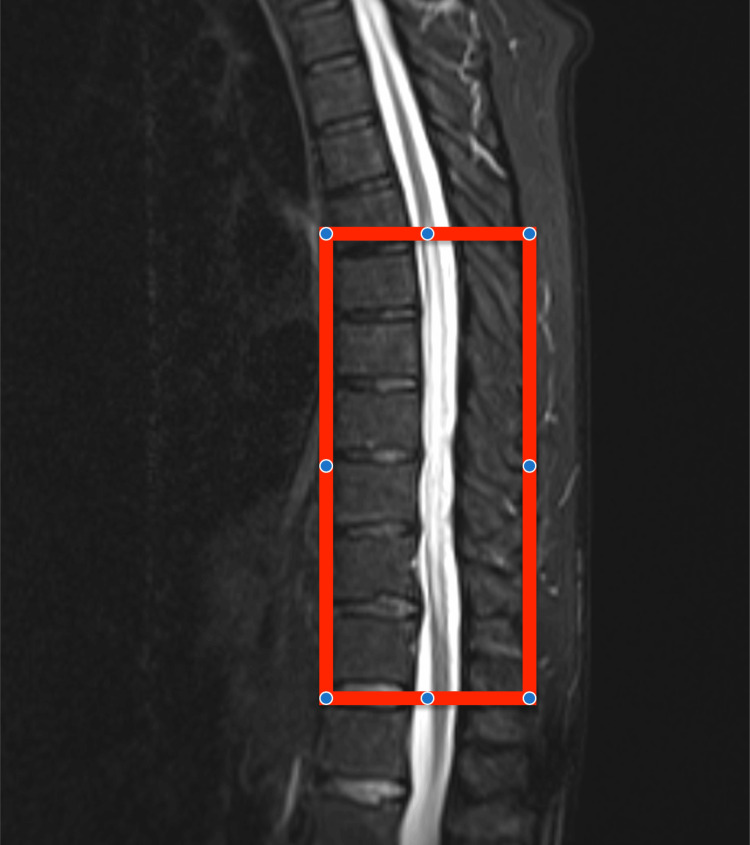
Initial MRI showing a longitudinally extensive T2 hyperintense lesion spanning T7-T11.
